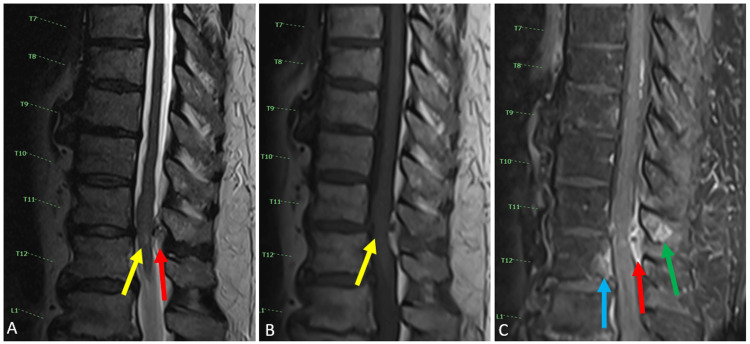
Sagittal MRI of the Thoracic Spine With and Without Contrast Sagittal MRI of the thoracic spine with T2 image (A), T1 image (B), and T1 post-contrast fat saturation image (C). Figure A demonstrates the expansion of the conus medullaris with an abnormal focus of T2 hyperintense signal (yellow arrow) with severe spinal canal stenosis caused by a heterogenous posterior epidural lesion (red arrow). Figure B demonstrates severe spinal canal stenosis (yellow arrow). Figure C demonstrates abnormal enhancement of the posterior epidural lesion (red arrow), which causes severe spinal canal stenosis. There is an abnormal enhancement of the posterior cortex of the T12 vertebral body (blue arrow) and an enhancement of the T11 spinous process (green arrow).
